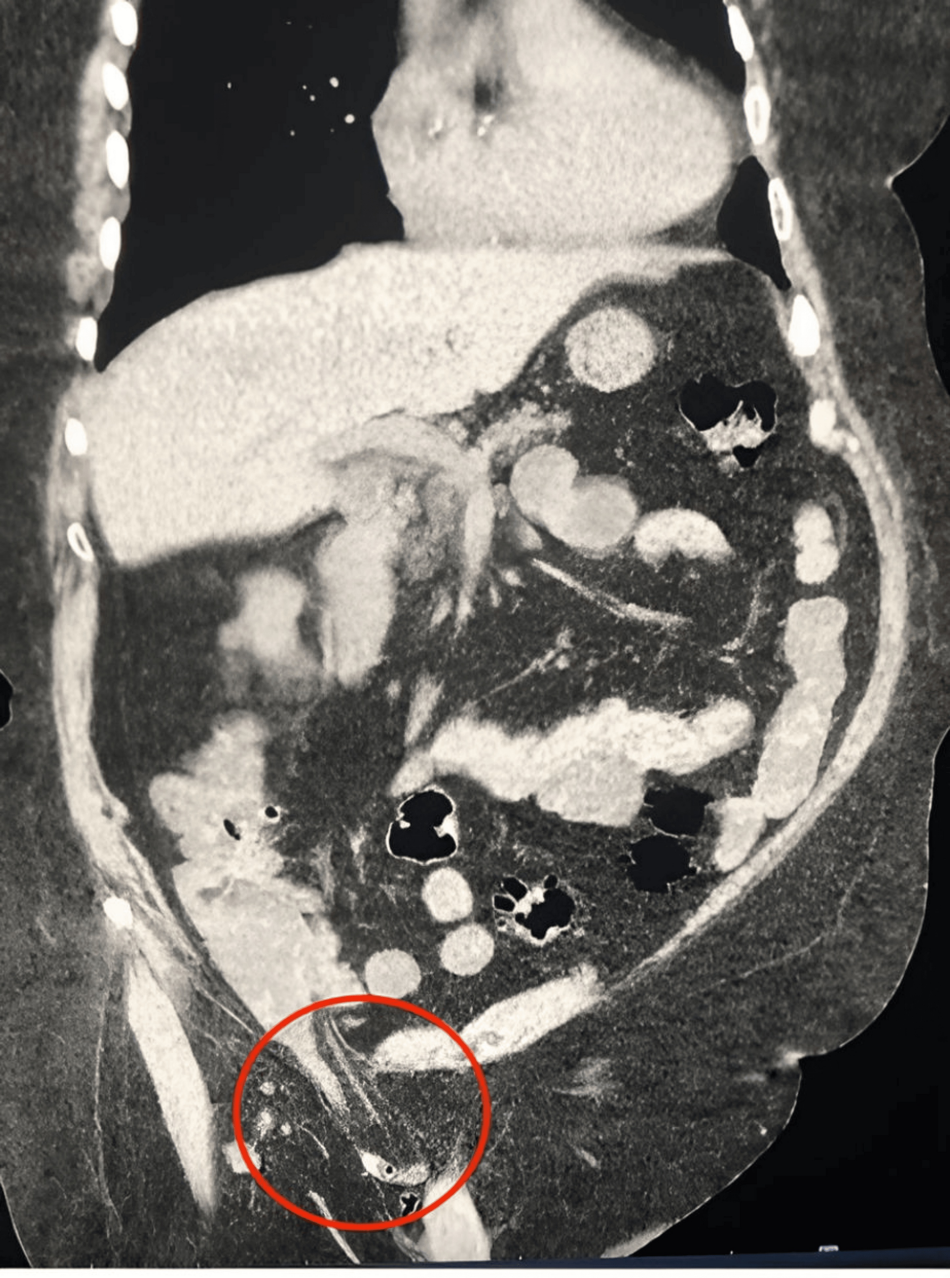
Coronal non-contrast CT of the abdomen showing a tubular structure arising from the cecum and mesenteric fat in the hernia sac (red circle).
